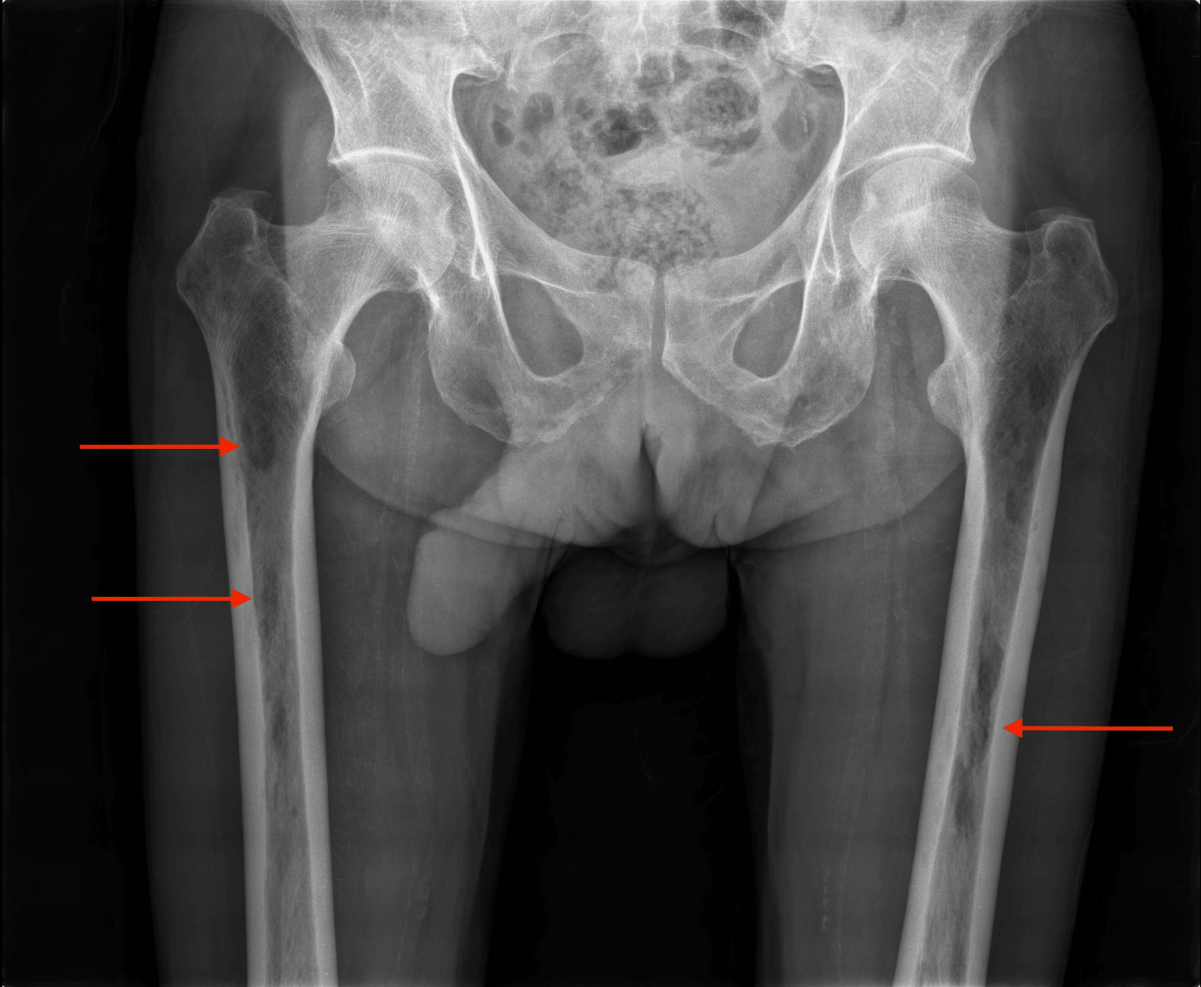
X-ray of the femur Right and left femur with osteolytic lesions (red arrows)
